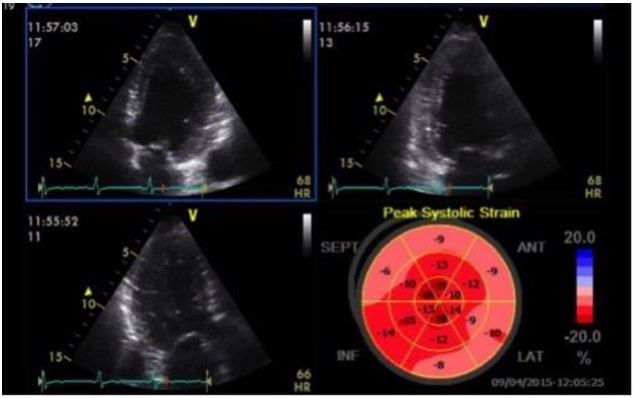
Follow-up echocardiogram of patient from Figure 4 24 hours after presentation. Quad view, Global longitudinal strain is now −13.5%. Anteroseptal, anterior and lateral wall dyskinesis has resolved following afterload reduction.
